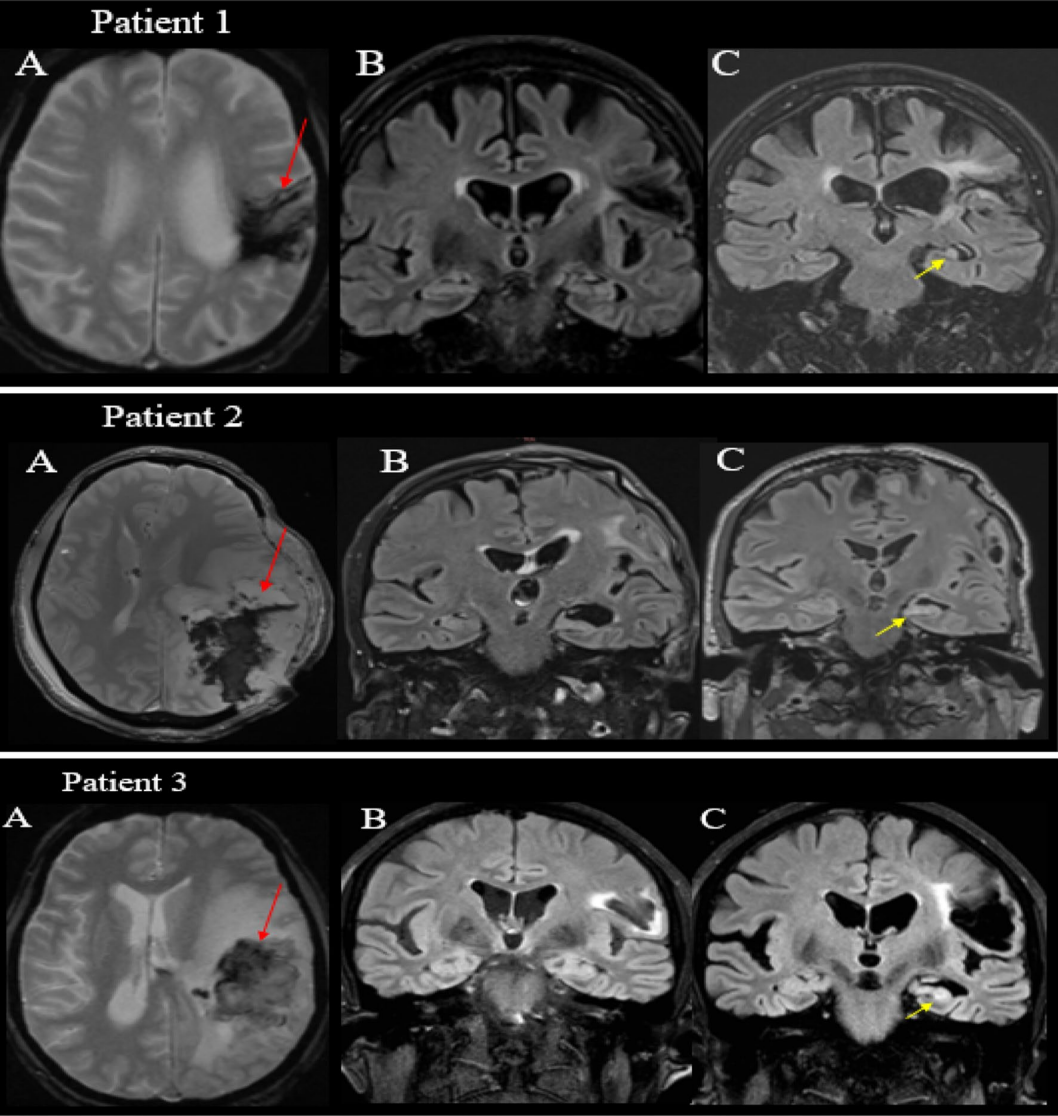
Cerebral MRI from the initial insult to the hippocampal sclerosis in patients 1, 2, and 3. A Axial SWI-MRI showing the acute hemorrhagic lesion (red arrow). B Coronal MRI FLAIR showing symmetric hippocampi at stroke onset. C Coronal MRI FLAIR showing hippocampal sclerosis after a minimum of 2 years (yellow arrow). *FLAIR* Fluid-attenuated inversion recovery, *SWI* Susceptibility weighted imaging
